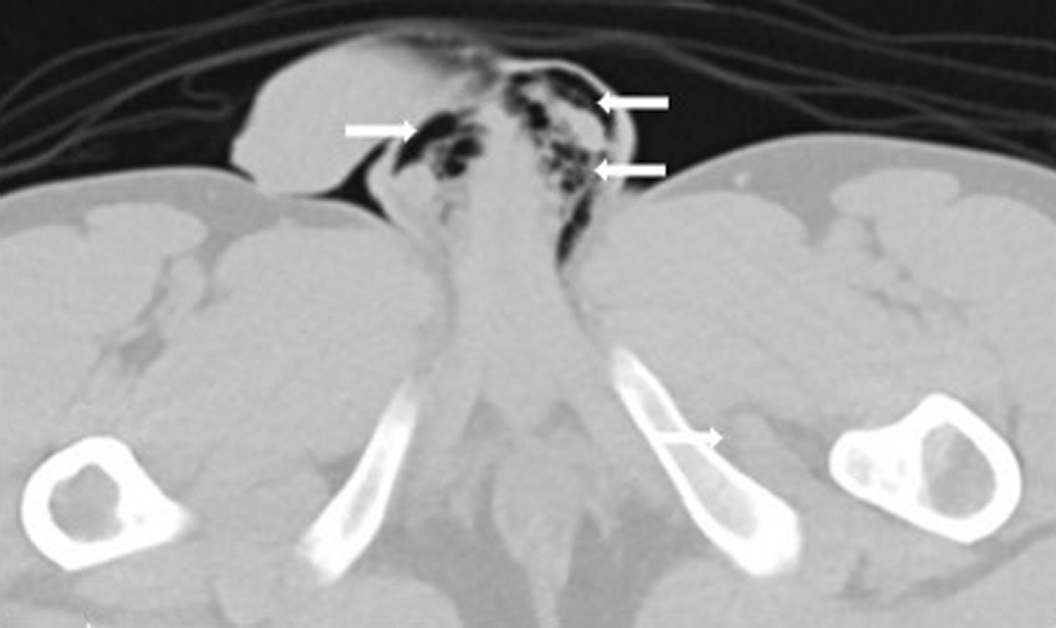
CT scan pelvis showing Pneumoscrotum.
